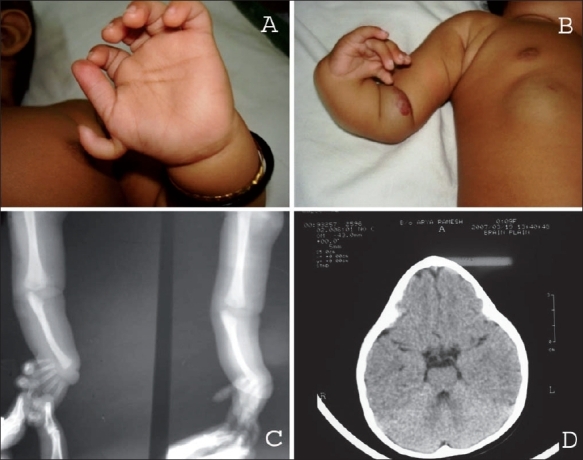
(A) Left hand showing an accessory thumb; (B) Right radial club hand and hemangiomas on the anterior aspect of the right elbow and the abdominal wall; (C) Anteroposterior and lateral view X-ray images of the right upper limb show the absent radius and the deformed short ulna in the right forearm; (D) CT scan of the brain showing normal brain parenchyma, fusion of the metopic suture with decrease in transverse diameter of the frontal region and anterior beaking in the mid frontal region (D). The sagital, the coronal and the lambdoid sutures are not fused
